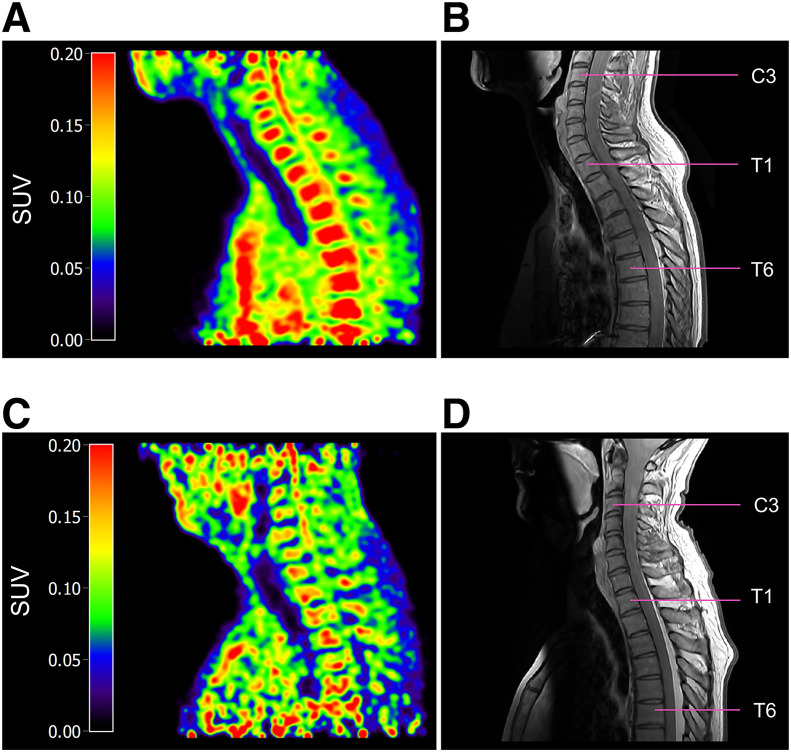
Sagittal [^11^C]MeDAS PET of cervical spinal cord of HC and MS patient (5 iterations, 5-mm gaussian filter). (A) [^11^C]MeDAS PET scan of spinal cord from C3 to T8 of HC. (B) Proton density–weighted MRI of spinal cord from C3 to T8 of same HC. (C) [^11^C]MeDAS PET scan of spinal cord from C1 to T6 of MS patient. (D) Proton density–weighted MRI of spinal cord from C1 to T6 of same MS patient.
